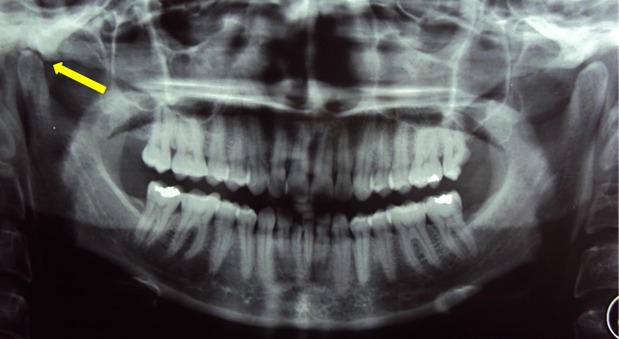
Orthopantomogram showing pinching space of the right TMJ
